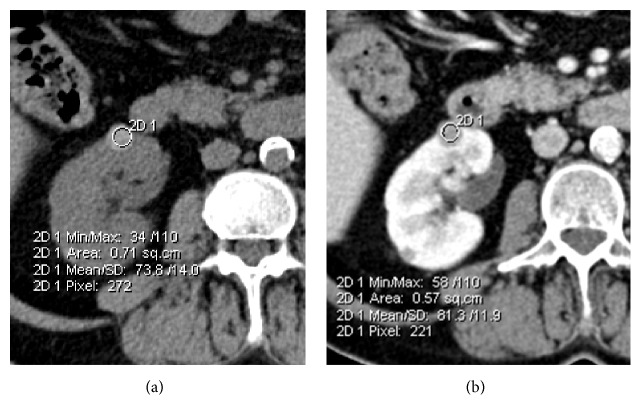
Benign hyperdense renal cyst. Axial CT-scan before (a) and after contrast medium administration (b). The region of interest (ROI) positioned on the small exophytic renal cyst shows high-density content (73 HU) without any significant increase in postcontrastographic study.
